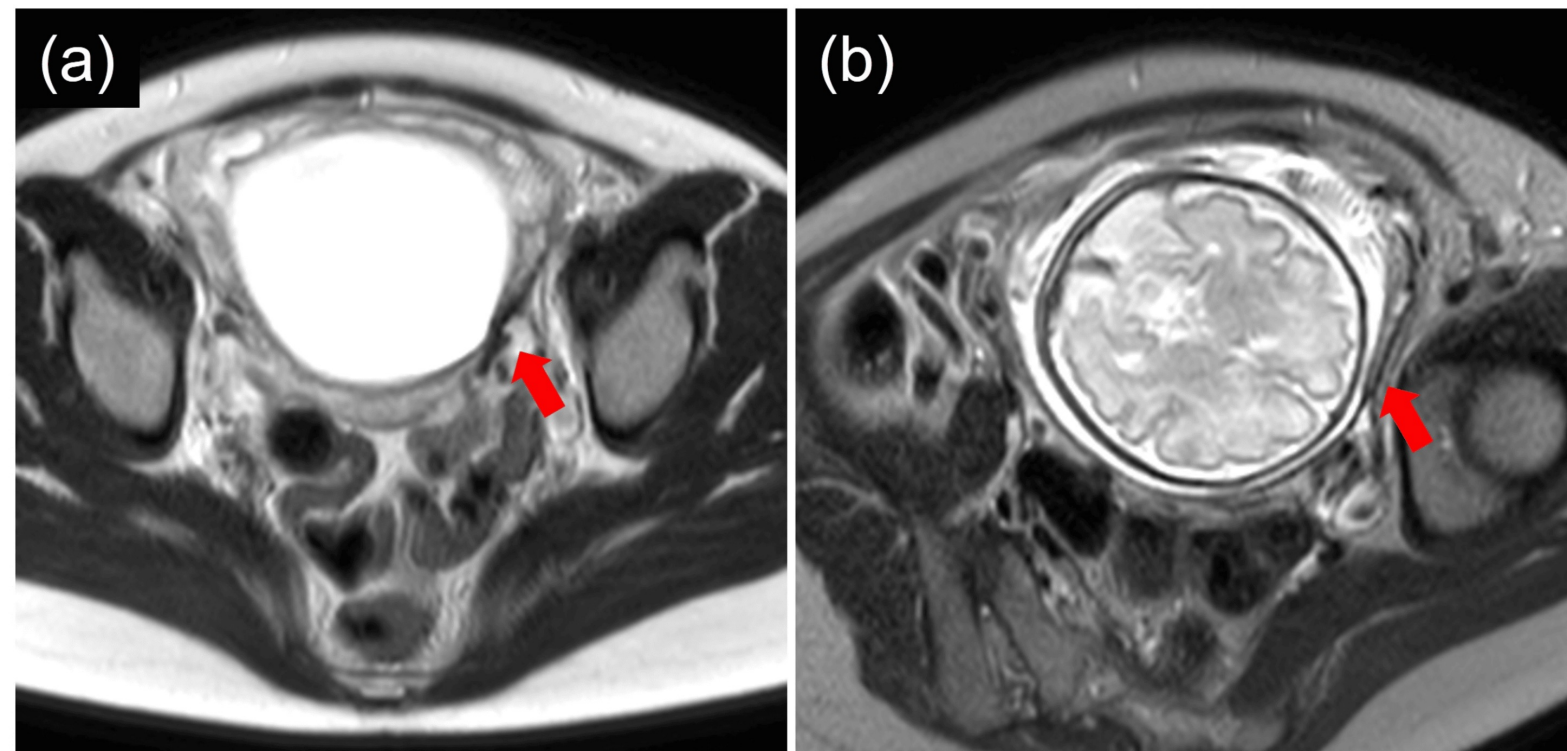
Magnetic resonance imaging during the current pregnancy. (a, b) Axial T2-weighted images at 24 (a) and 31 (b) weeks of gestation. Thinning of the myometrium is observed on the left side of the lower uterus. Although the normal uterine myometrium appears indistinct, no clear signs of a silent rupture are evident. The red arrows indicate the uterine rupture scar.
